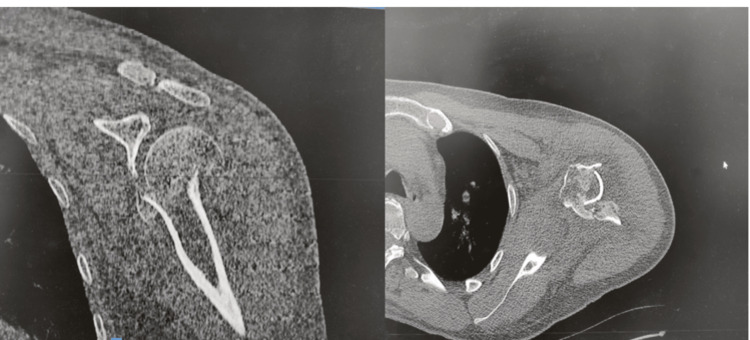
CT images demonstrating a fracture of proximal humerus Image Credits: First Author
